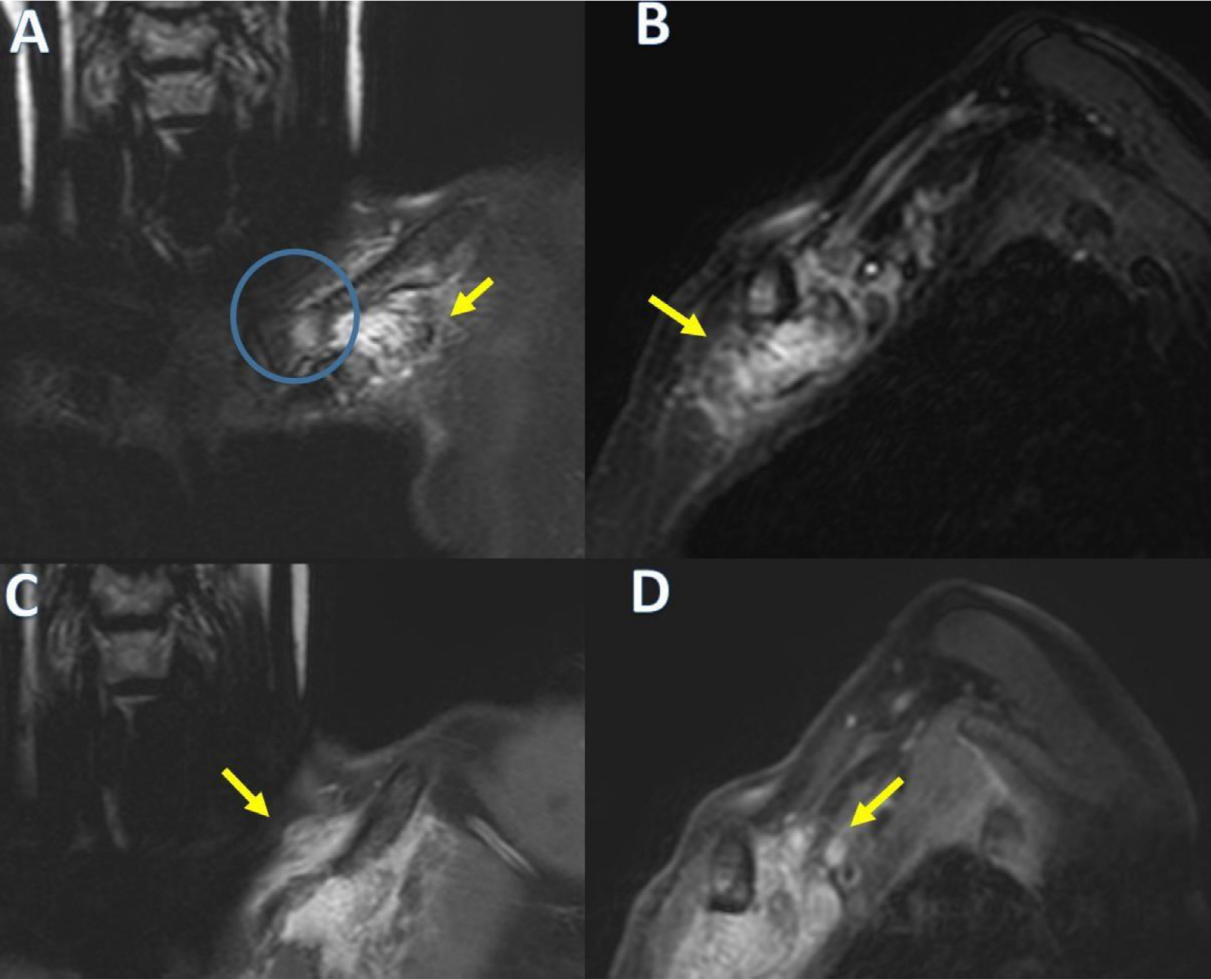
Magnetic resonance imaging (MRI) using T2 Fat Sat (A in sagittal plane and B in coronal plane), and post contrast T1 Fat Sat (C in sagittal plane and D in coronal plane) weighted sequences show a soft tissue mass predominantly hyperintense in T2 FatSat-weighted sequences (yellow arrows) with extensive and diffuse contrast enhancement that extends to the adjacent soft tissue. Mild edema and post contrast enhancement of the adjacent clavicle (green arrow) is also observed.
